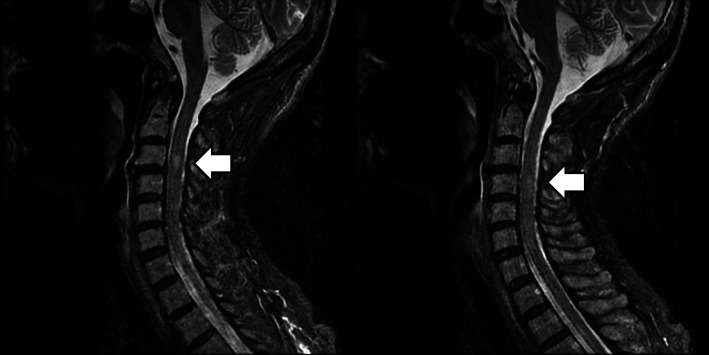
T2 weighted cervical magnetic resonance imaging (MRI) without contrast. Cervical spine MRI showing mild increase in the T2-signal intensity of the cervical cord at the C3–C4 levels
